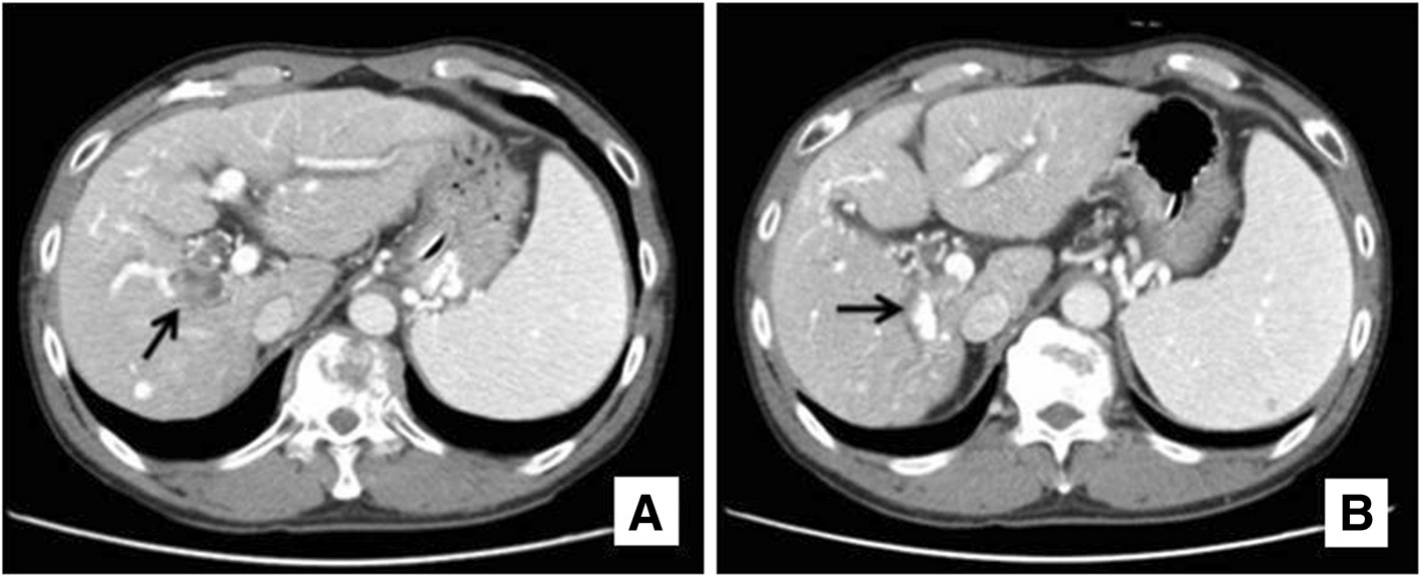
Computed tomography. (A) A thrombus was noted in the posterior branch of right portal vein (arrow). (B) Dilatation of intrahepatic duct (arrow) adjacent to a partially lipiodolized nodule suggested direct invasion of the tumor into the surrounding intrahepatic duct in the portal-venous phase.
